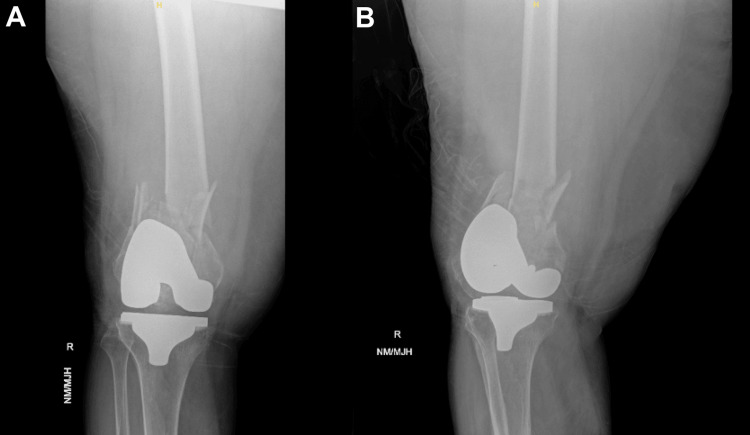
Initial radiographs of the right knee demonstrating a periprosthetic femoral fracture (A) Anteroposterior and (B) lateral radiographs of the right knee demonstrating a comminuted periprosthetic distal femoral fracture (Arbeitsgemeinschaft für Osteosynthesefragen (AO)/Orthopaedic Trauma Association (OTA) 33A3) located proximal to a well-fixed TKA implant. The fracture extends proximally into the diaphysis with displacement and fragmentation.
